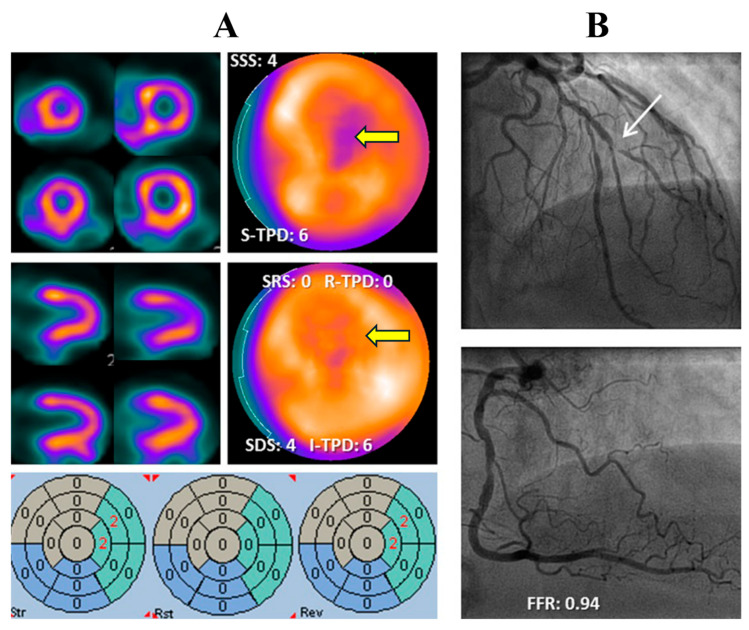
Automation in Myocardial Perfusion Imaging (MPI). Stress ((A), top row) and rest ((A), middle row) single-photon emission computed tomography (SPECT) images demonstrating a reversible perfusion defect in the mid-to-apical anterolateral wall (yellow arrows). Automated quantification maps ((A), bottom row) highlight perfusion deficits using a standardized 17-segment model. (B). Invasive coronary angiography showing subtotal stenosis of a diagonal branch (white arrow) with corresponding physiological assessment (FFR: 0.94). Adapted from Driessen RS et al. [34].
